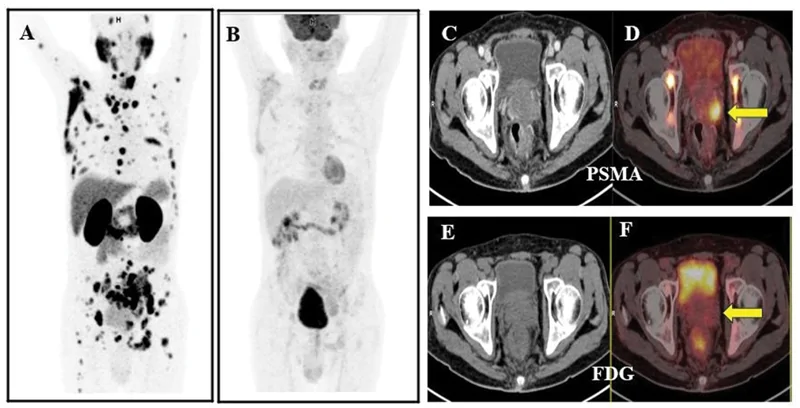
Discordance between gallium-68 (Ga-68) prostate-specific membrane antigen (PSMA) positron emission tomography/computed tomography (PET/CT) and F-18 fluorodeoxyglucose (FDG) PET/CT. (
